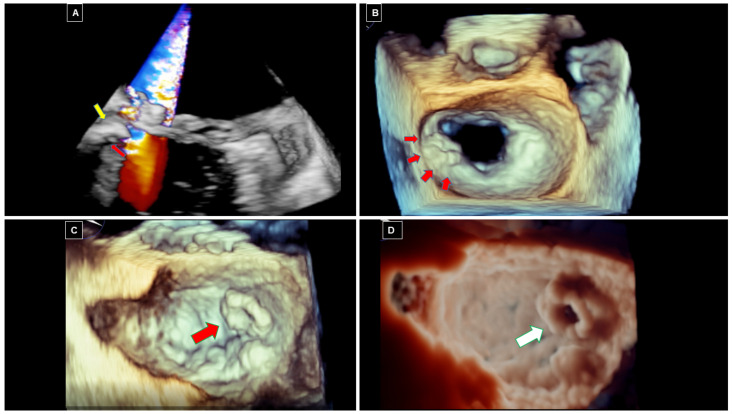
Three-dimensional transesophageal echocardiography details of ruptured CCMA. (A) shows color-Doppler assessment of ventricular-atrial fistulization and relationship with ruptured medial annulus and commissure. (B,C) show volume rendering surgical views of mitral valve with ruptured CCMA at lateral (B) and medial (C) portion of mitral annulus in two different patients. (D) shows the same image of (C), but highlighted by transilluminating mode, clearly demonstrating a hole in the lesion appearing as a crater.
